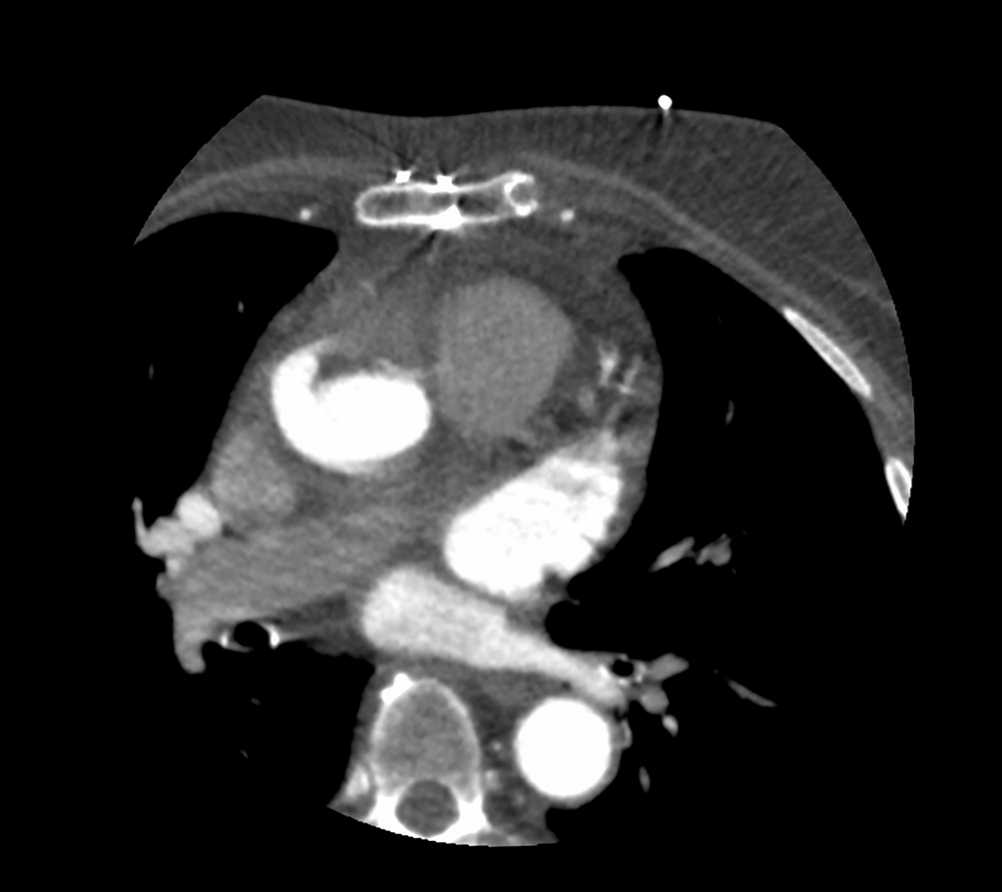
Axial slice of CT angiography. The anterior wall of the graft is irregularly thickened with a hypodense component extending into the lumen.
